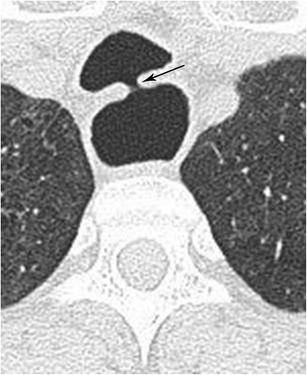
Tracheoesophageal Fistula. CT of the chest in lung window in axial plane demonstrates communication between the trachea and oesophagus (arrow) secondary to prolonged endotracheal tube intubation
